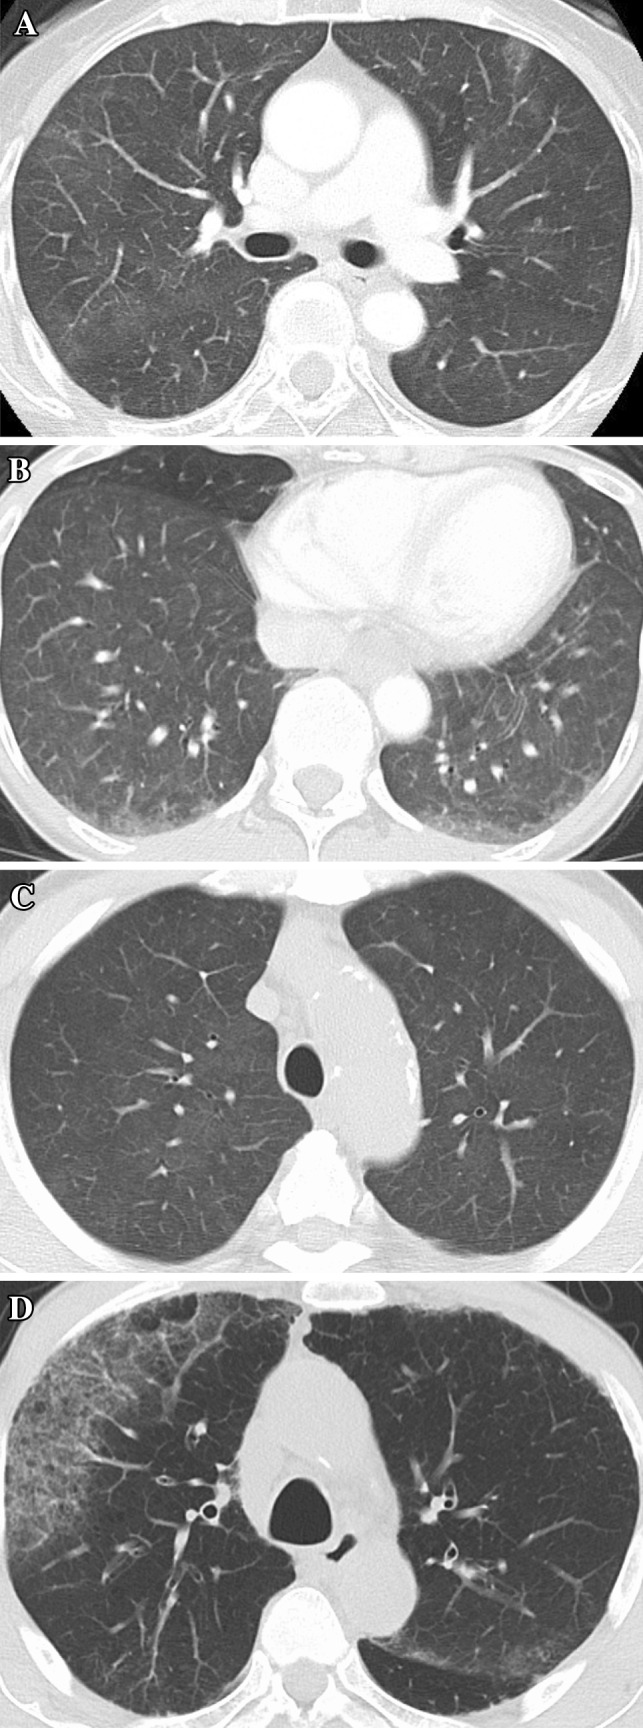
a Chest CT scans of a 65-year-old female non-smoker with lung and liver metastases at 8 weeks. b Chest CT scans of a 64-year-old female non-smoker with locally advanced cancer at 12 weeks. c Chest CT scans of a 76-year-old male ex-smoker with lung metastasis at 4 weeks. d Chest CT scans of a 70-year-old male ex-smoker with liver metastasis at 6 weeks
